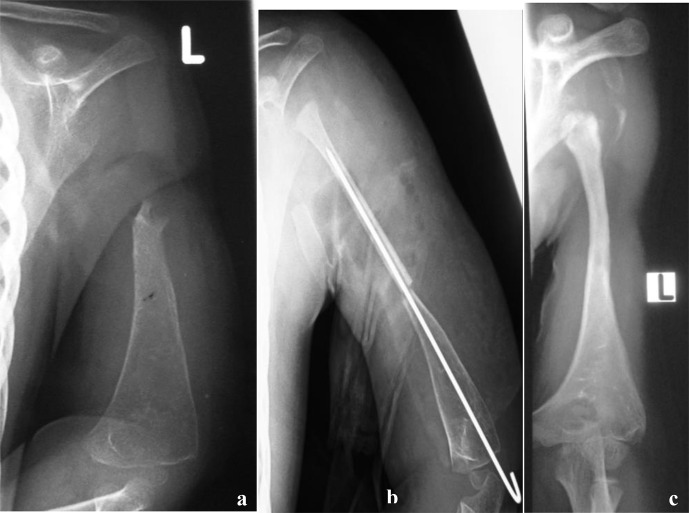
(a) Radiograph at presentation of a 3-year-old child referred for a possible shoulder disarticulation; (b) a proximal fibular epiphysis was used for bone and joint reconstruction; (c) radiograph taken 6 years later demonstrates reconstruction of the proximal half of the humerus, with an expanded and more spherical pseudo-humeral head.
